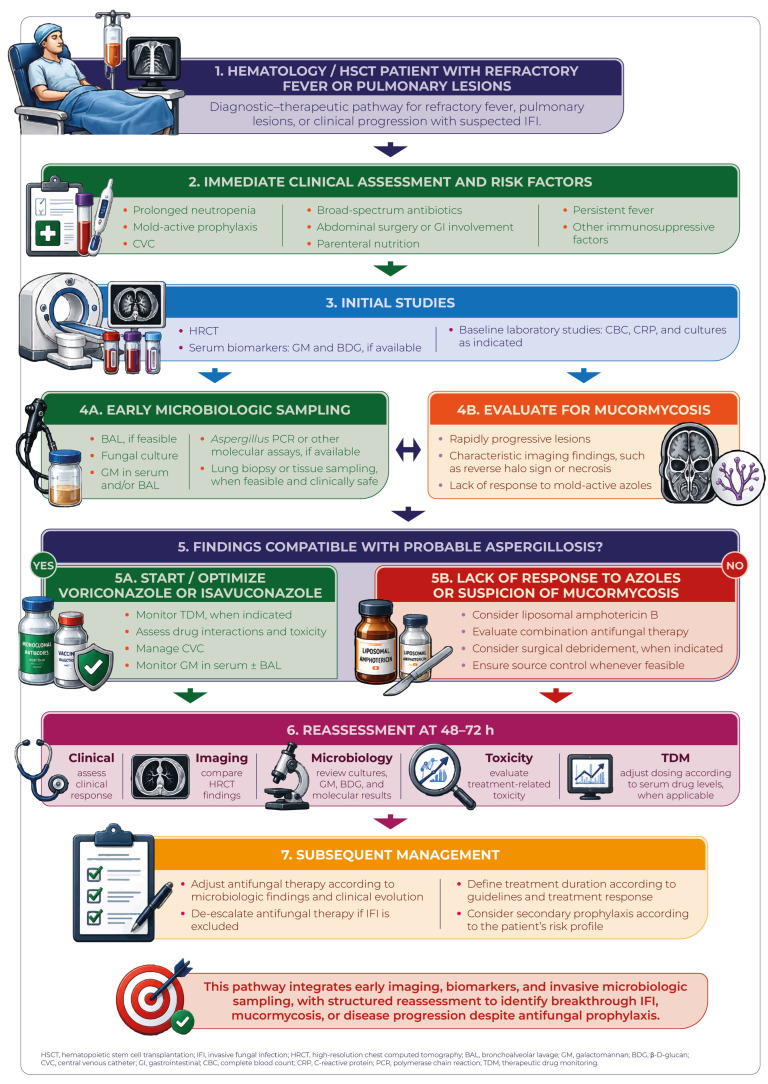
Diagnostic algorithm for hematology and hematopoietic stem cell transplant patients with suspected invasive fungal infection. This algorithm provides a practical diagnostic–therapeutic pathway for patients with hematological malignancies or HSCT who develop refractory fever, new pulmonary lesions, or clinical progression despite antibacterial therapy or mold-active prophylaxis. The pathway emphasizes early HRCT, contextual interpretation of fungal biomarkers, and prompt microbiological sampling, including BAL or tissue biopsy when feasible and safe. Because breakthrough infection may reflect pathogens not covered by prior prophylaxis, progressive lesions, necrosis, suggestive imaging, or poor response to mold-active azoles should prompt active evaluation for mucormycosis and other non-Aspergillus molds. Reassessment at 48–72 h integrates clinical response, radiologic evolution, microbiology, toxicity, and therapeutic drug monitoring to guide treatment optimization, escalation, de-escalation, or secondary prevention.
